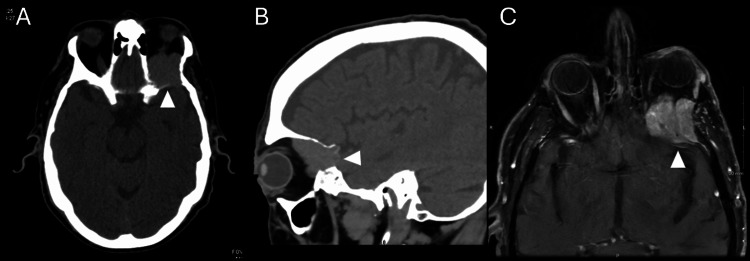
Head CT and MRI demonstrate left sphenoid bone metastasis extending into the posterior orbit
